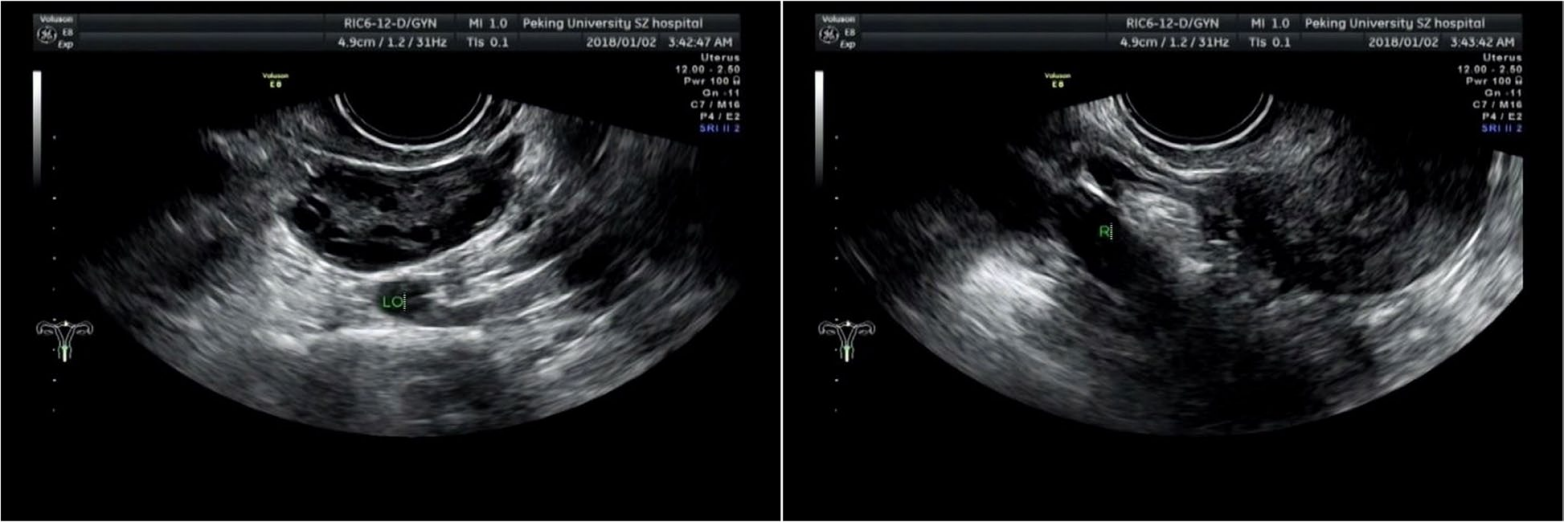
The transvaginal ultrasound image of Case 2: Transvaginal ultrasound image showed PCO (polycystic ovary)changed in the left ovary, and the right ovary was unclear
